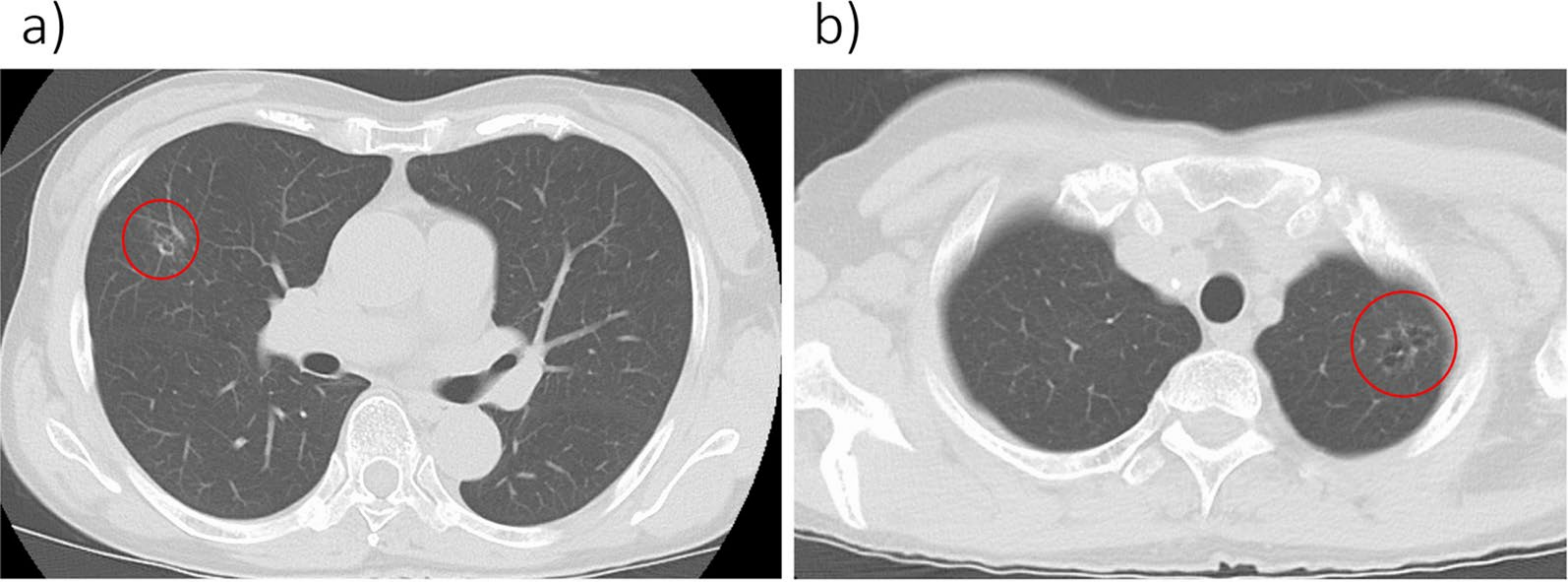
Computed tomography findings of the lung tumor. a A frosted glass shadow is observed in the middle lobe of the right lung. b A frosted glass shadow is observed in the upper lobe of the left lung
